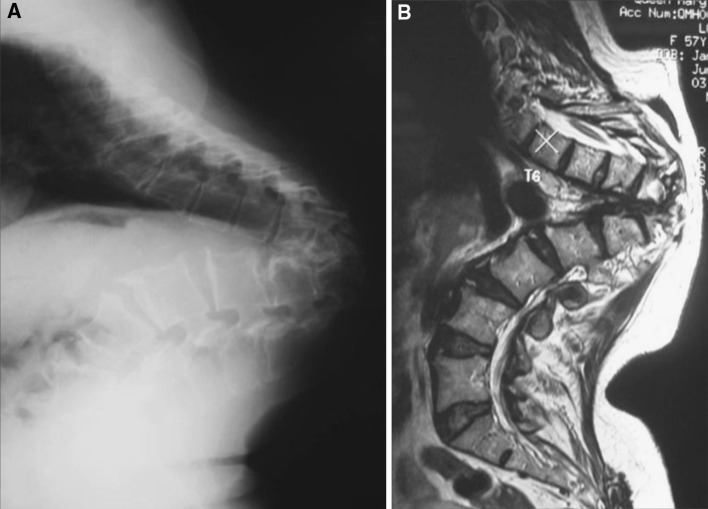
Late-onset Pott’s paraplegia with healed disease. a Severe kyphosis at the lower thoracic region. b MRI showed no evidence of reactivation of infection
